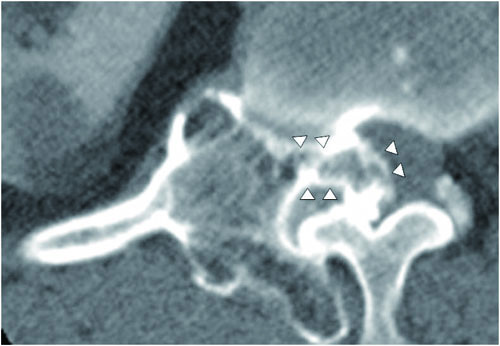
Axial CT images. The images show an osseous mass arising from the head of the right 12th rib at the costovertebral junction with obliteration of the neural foramen and intraspinal extension.
